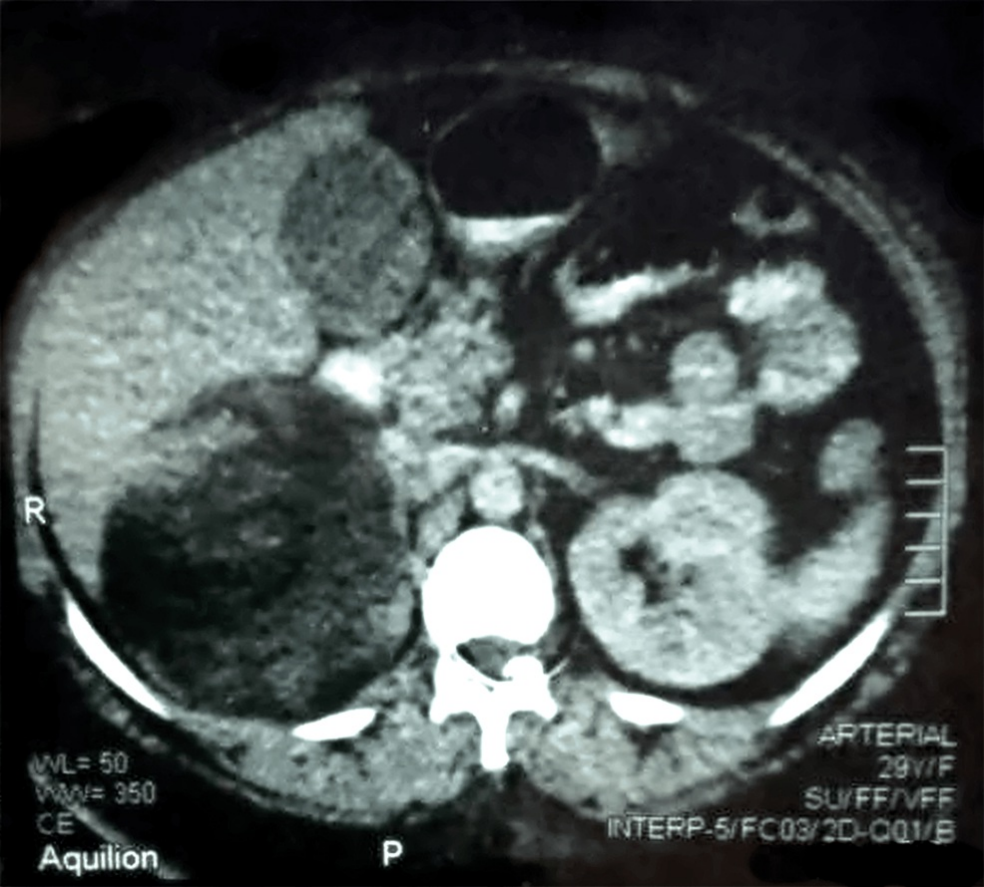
Computed tomography of the abdomen, transverse section with oral and intravenous contrast enhancement A large inhomogeneous lesion is seen dorsal to the liver. The mass measures up to 15 cm and displaces the liver anteriorly and the neighboring organs medially. The study shows no evidence of local invasion, enlarged lymph nodes, contralateral masses, or liver metastases.
